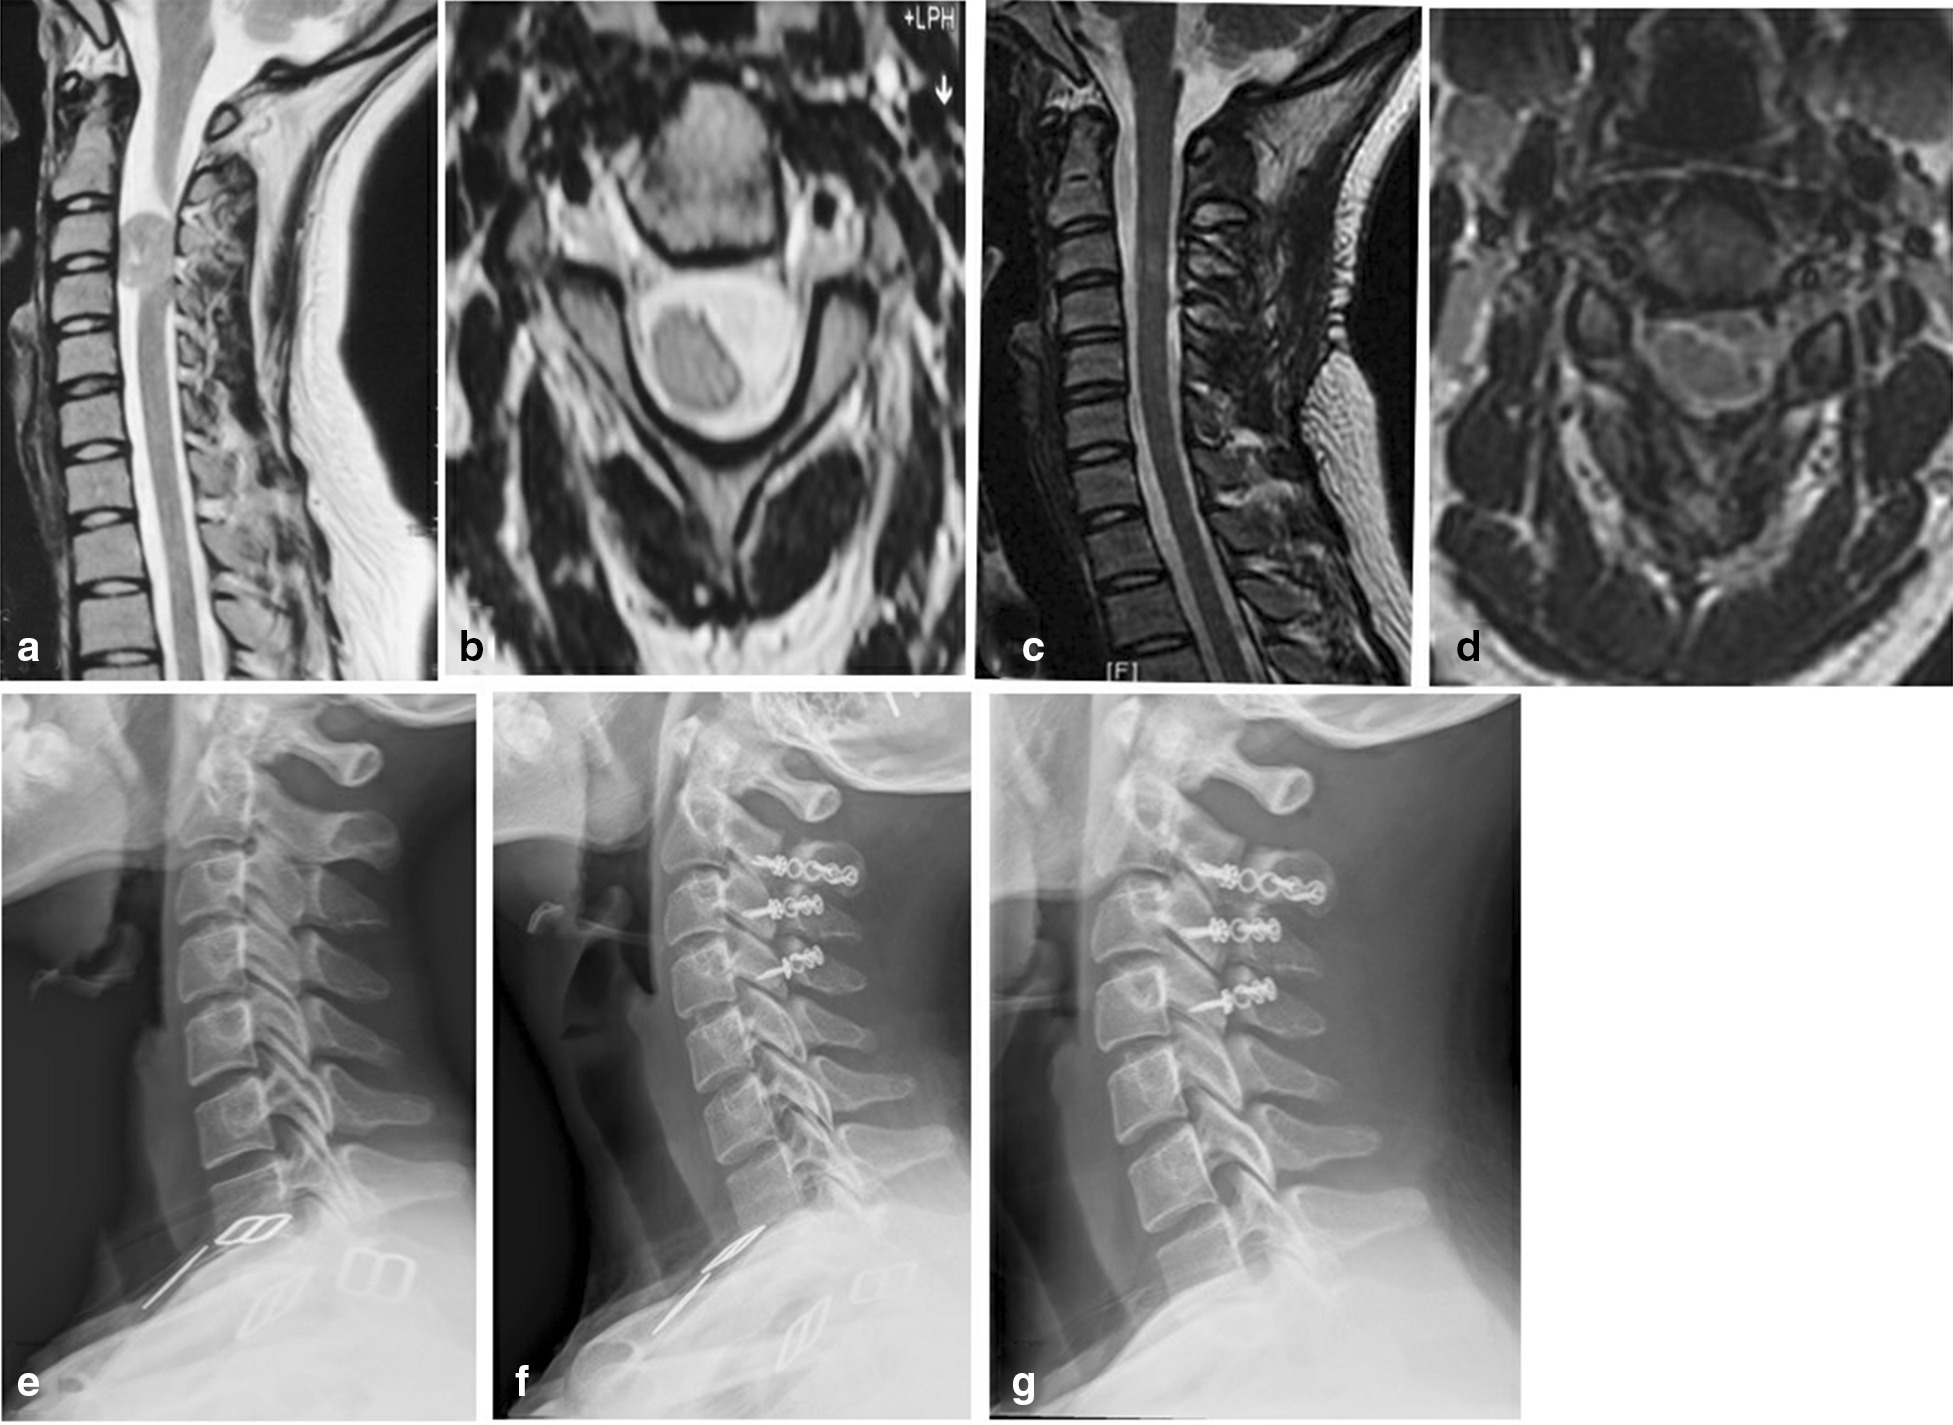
Case 3. The schwannoma at the C3-4 level. a, b Prior to surgery, MRI identified that the C3-4 spinal space was occupied by the tumor. c, d At 12 months after the surgery, no intraspinal scar adhesions or restenosis were identified by MRI. e Prior to surgery, cervical X-ray examination was performed under the upright conditions. f, g At 12 months and 24 months after the surgery, X-ray examination indicated no fixation transposition or fracture, cervical instability or kyphosis
